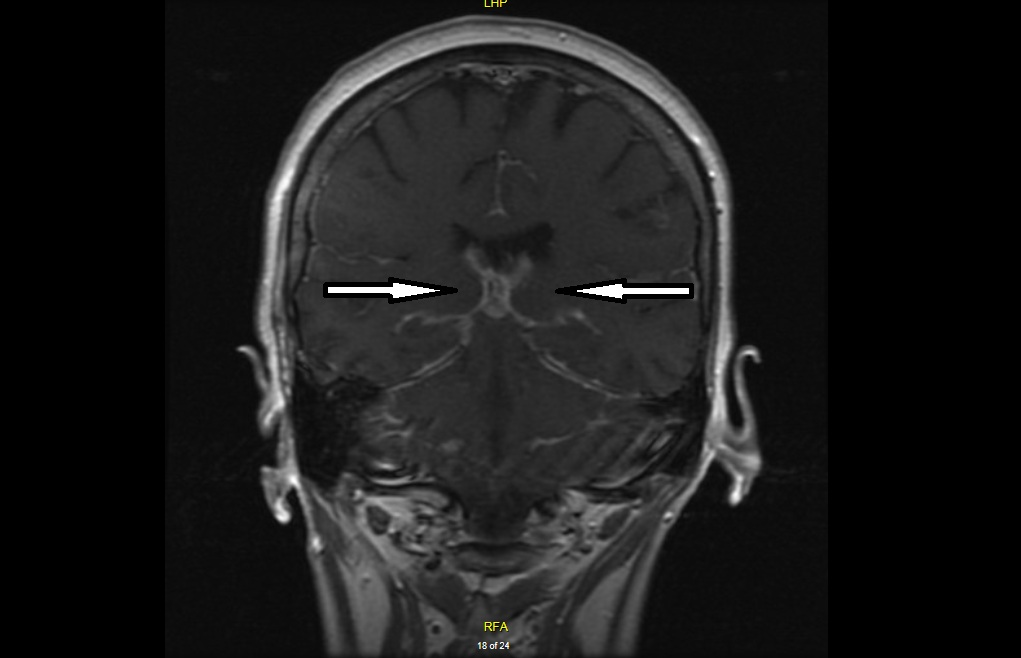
MRI of the brain with contrast (coronal section) showing widespread leptomeningeal enhancement (arrows) raising suspicion for leptomeningeal carcinomatosis.
